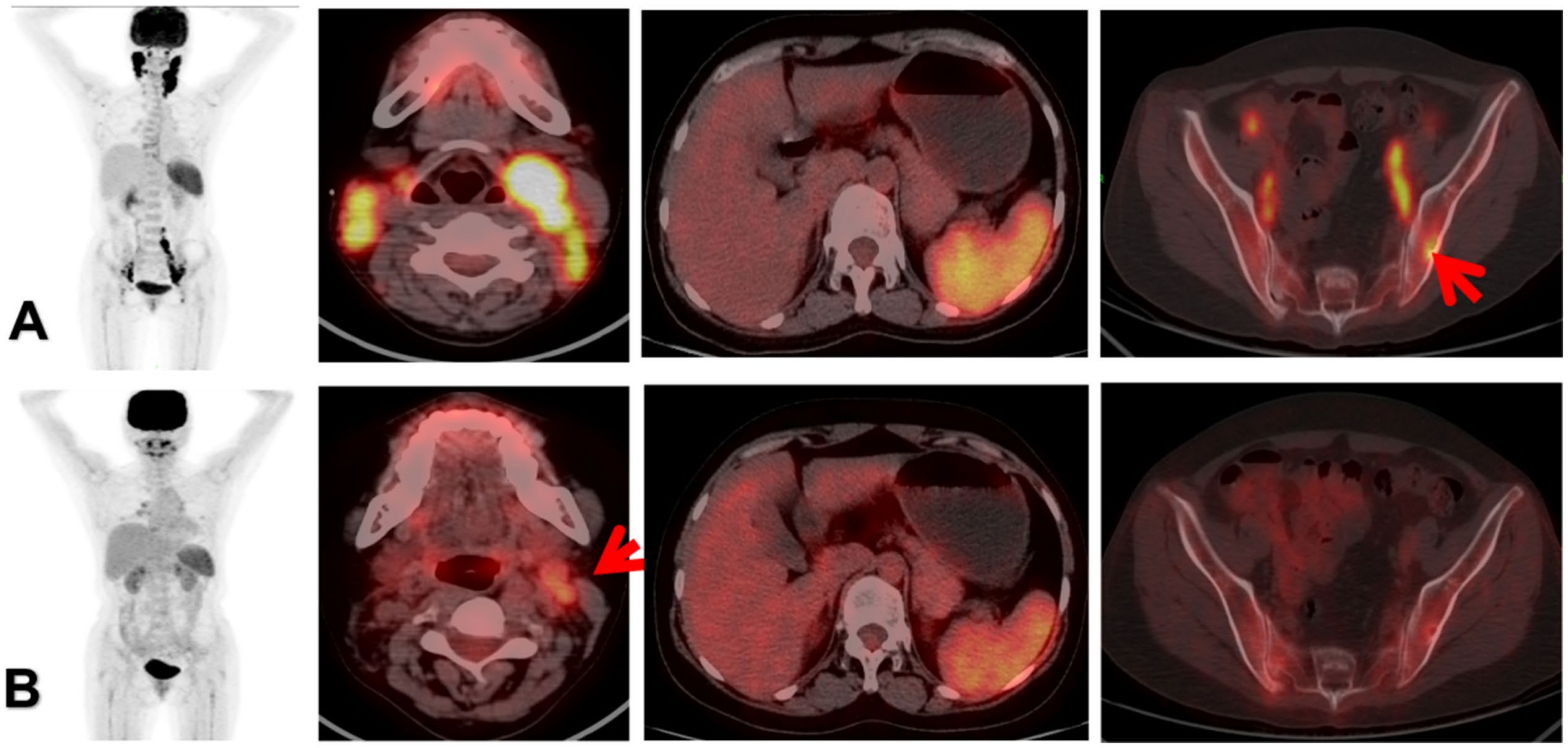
The patient was a 35-year-old female. Panel (A) was the 18F-FDG PET/CT before treatment, multiple enlarged lymph nodes were found in the bilateral neck, iliac vessels and retroperitoneum, with increased radioactive uptake. Among them, the SUVmax of the left cervical lymph nodes was 12.8, the MTV was 18.7, the TLR was 7.5, and the TLG was 152.3. Local bone destruction occurred in the left iliac bone, with increased metabolism, and the SUVmax was 4.8. The morphology and size of the spleen are normal, with increased diffuse radioactive uptake, and the SUVmax was 7.6. The clinical stage was stage III, classified as MS-LCH RO+, and BRAF V600E was positive. Panel (B) was reexamined after the treatment with the VP protocol. 18F-FDG PET/CT showed that the bilateral cervical lymph nodes were significantly reduced, the metabolism was decreased, and the metabolism of the spleen and the left iliac bone was significantly reduced, suggesting a significant therapeutic effect. However, a recurrence occurred during the follow-up visit half a year later. The red arrow of (A) indicated focal infiltration of the left ilium. The red arrow of (B) indicated that there was still mild FDG uptake in the left cervical lymph nodes, suggesting the presence of partial tumor activity.
